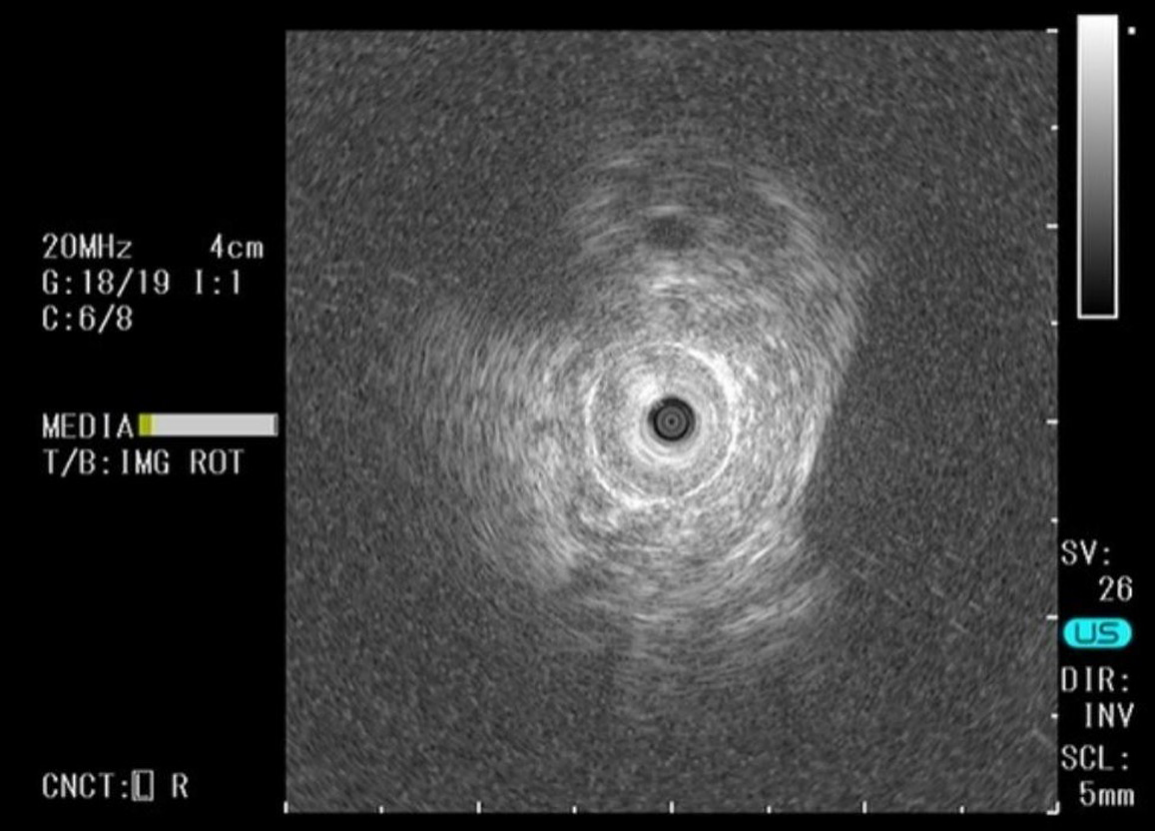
Intraductal ultrasonography showed a homogeneous circumferential thickening (iso‐to hyperechoic) of the bile duct wall at the site of the stenosis was observed.
